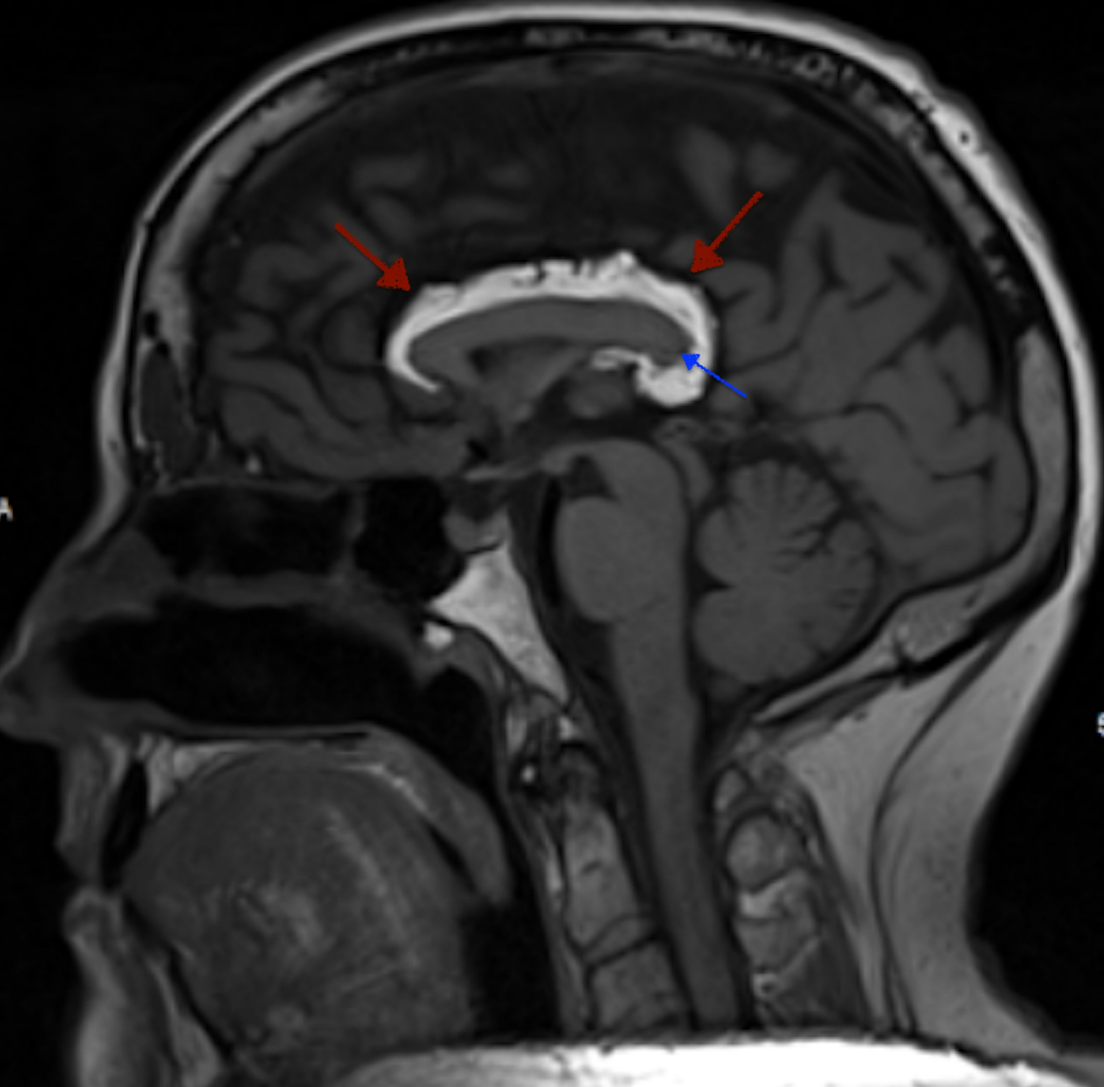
Sagittal pre-contrast T1-weighted MRI of the brain Sagittal pre-contrast T1-weighted MRI of the brain demonstrates a hyperintense lesion (red arrows) along the anterior and posterior aspects of the superior margin of the corpus callosum, curving around the splenium. Additionally, there is associated splenial hypoplasia (blue arrow) of the corpus callosum.
